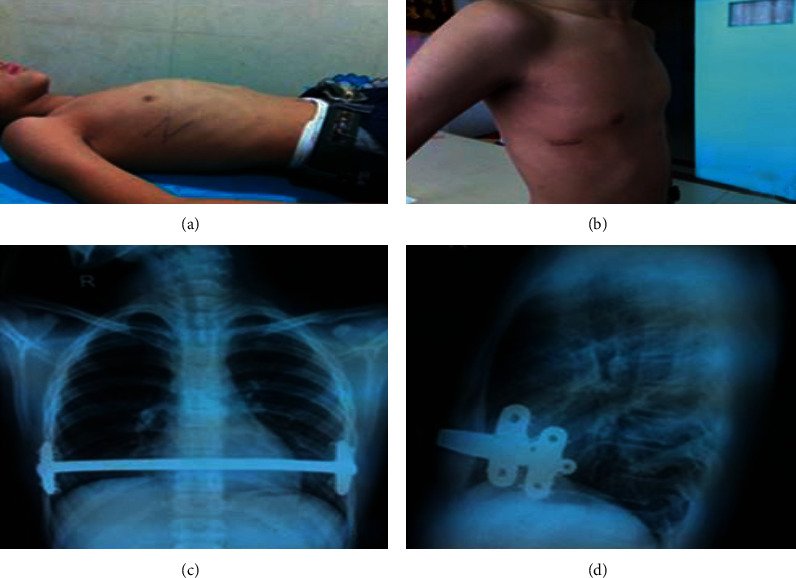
Minimally invasive sternal depression procedure for pectus carinatum: (a) preoperative appearance, (b) postoperative appearance and the incision locations, (c) postoperative frontal chest X-ray film, and (d) postoperative lateral chest X-ray film.
